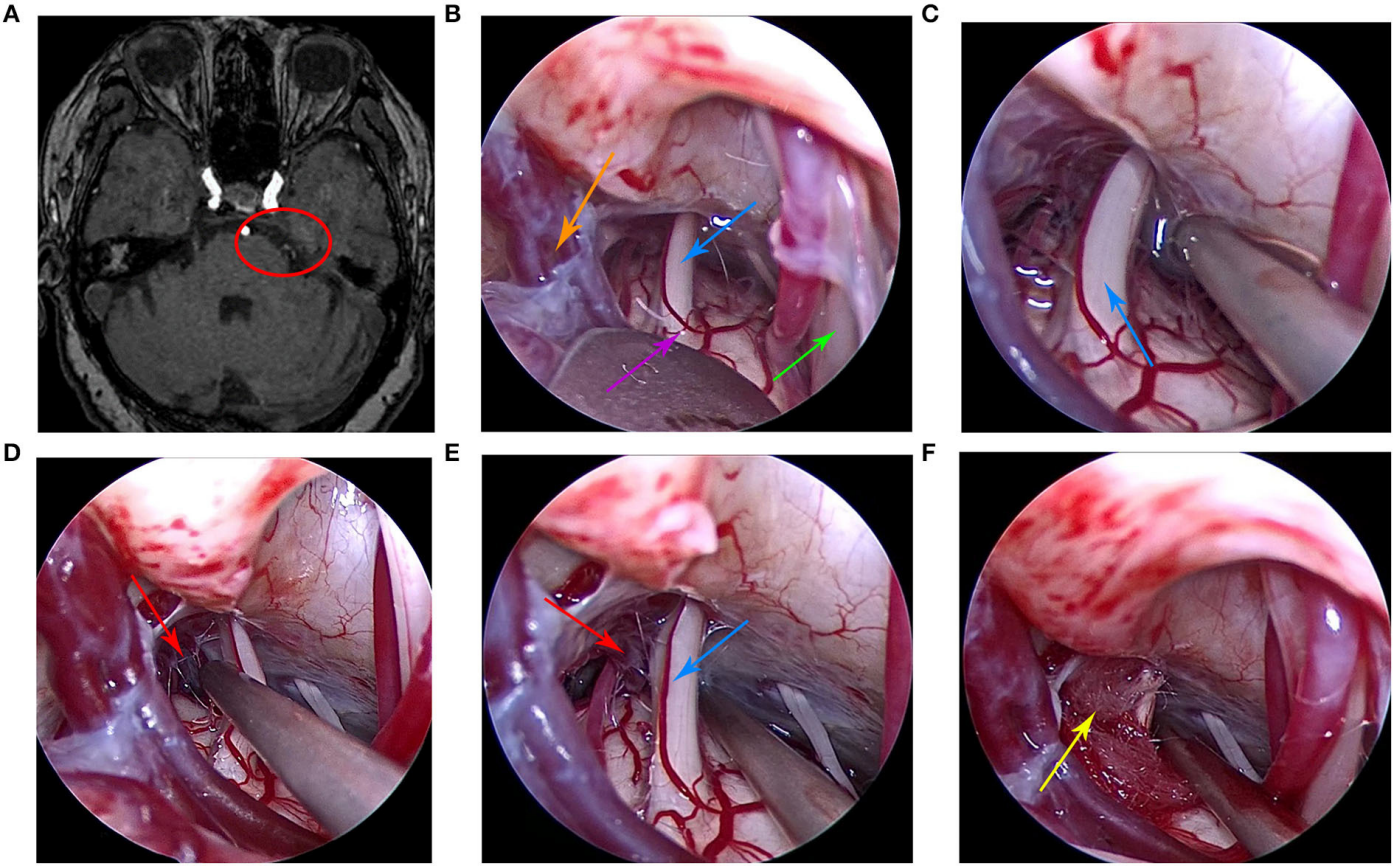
(A) Preoperative head magnetic resonance imaging of the patient. (B) Under the endoscope, the arachnoid was explored along the outer and upper margins of the cerebellum and subsequently cut to fully expose the trigeminal nerve. (C) At first, no obvious offending vessels were found. (D) After careful exploration, we found the offending small vein and arachnoid adhesions. (E) The petrosal vein branch and trigeminal nerve were clearly visible under the endoscopic view. (F) The Teflon pad was placed between the trigeminal nerve and the petrosal vein branch under the endoscopic view. The red circle indicates that the trigeminal nerve was compressed by the petrosal vein branch, the purple arrow indicates the brainstem, the orange arrow indicates petrosal vein, the red arrow indicates the petrosal vein branch, the blue arrow indicates the trigeminal nerve, the green arrow indicates the facial nerve, and the yellow arrow indicates the Teflon pad.
